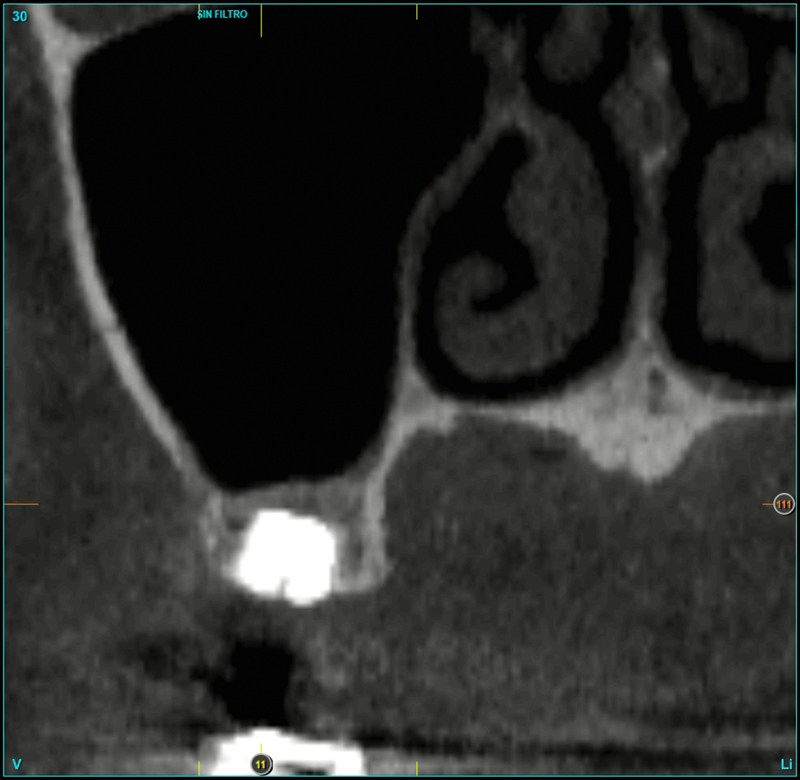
Cone-beam computed tomography image. Five months after the 4.5-length “temporary” implant placing. Three mm of bone gain over the implant apex could be observed.
